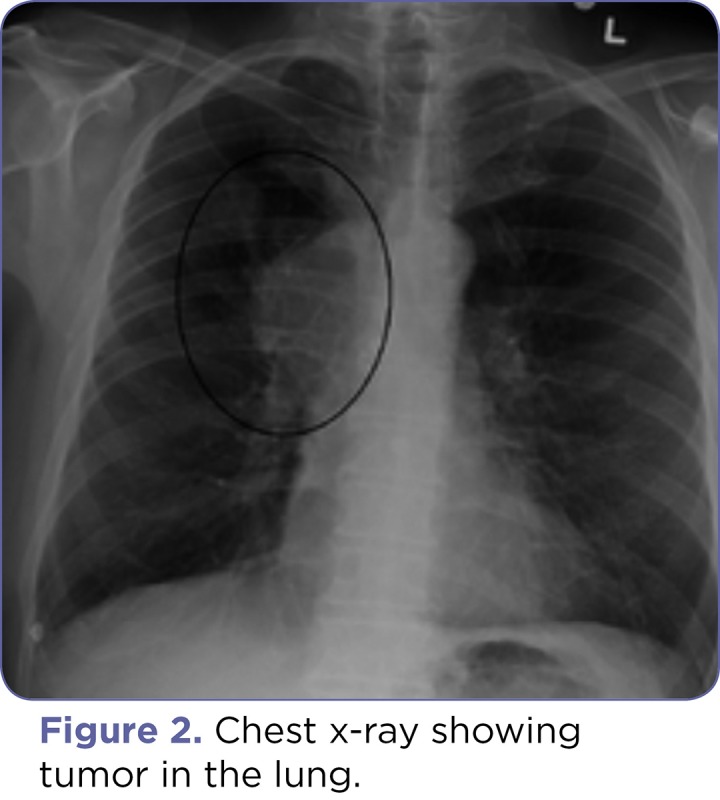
Figure 2. Chest x-ray showing tumor in the lung.
